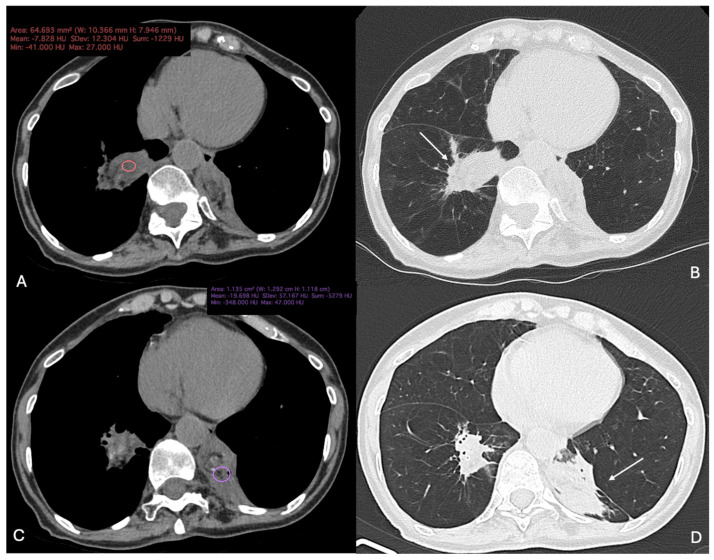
(A,B) Axial non-contrast HRCT images in mediastinal (A) and lung (B) windows in a woman with chronic aspiration of nasal allergy spray depict a mass-like consolidation without air bronchogram in the lateral and posterior segments of the right lower lobe, characterized by irregular spiculated margins (arrow in (B)). The consolidation shows negative attenuation values (mean −8 HU), indicating intralesional fat (red circle in (A)). Despite the spiculated morphology, lung biopsy confirmed the diagnosis of lipoid pneumonia. (C,D) Axial non-contrast HRCT images in mediastinal (C) and lung (D) windows in a patient with chronic aspiration of chemical lubricant reveal a mass-like consolidation involving the superior and basal segments of the left lower lobe (arrow in (D)). Mean attenuation measures −20 HU, consistent with lipid content within the lesion (purple circle in (C)).
